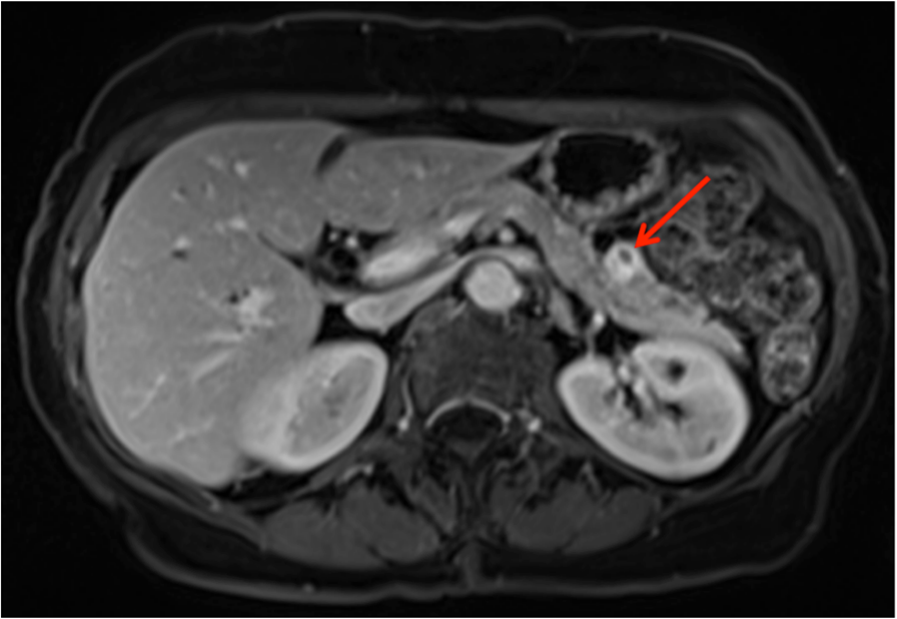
Axial venous phase MRI showing an enhancing 1.5 cm cystic mass consistent with pancreatic neuroendocrine tumor in the tail of the pancreas (*red arrow*)
